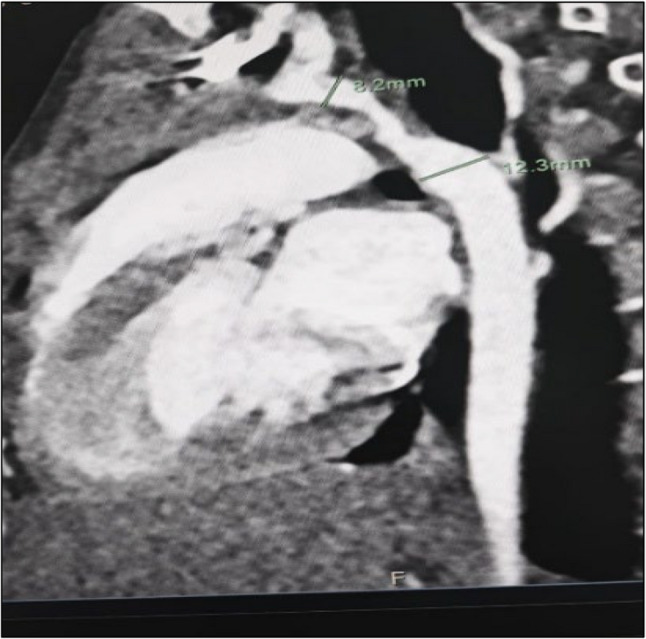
CT aortography (sagittal view) demonstrating severe long-segment coarctation of the aorta. The narrowest segment measures 2.2 mm (upper measurement), with proximal aortic diameter of 12.3 mm (lower measurement), showing marked discrepancy between pre-stenotic and stenotic segments
